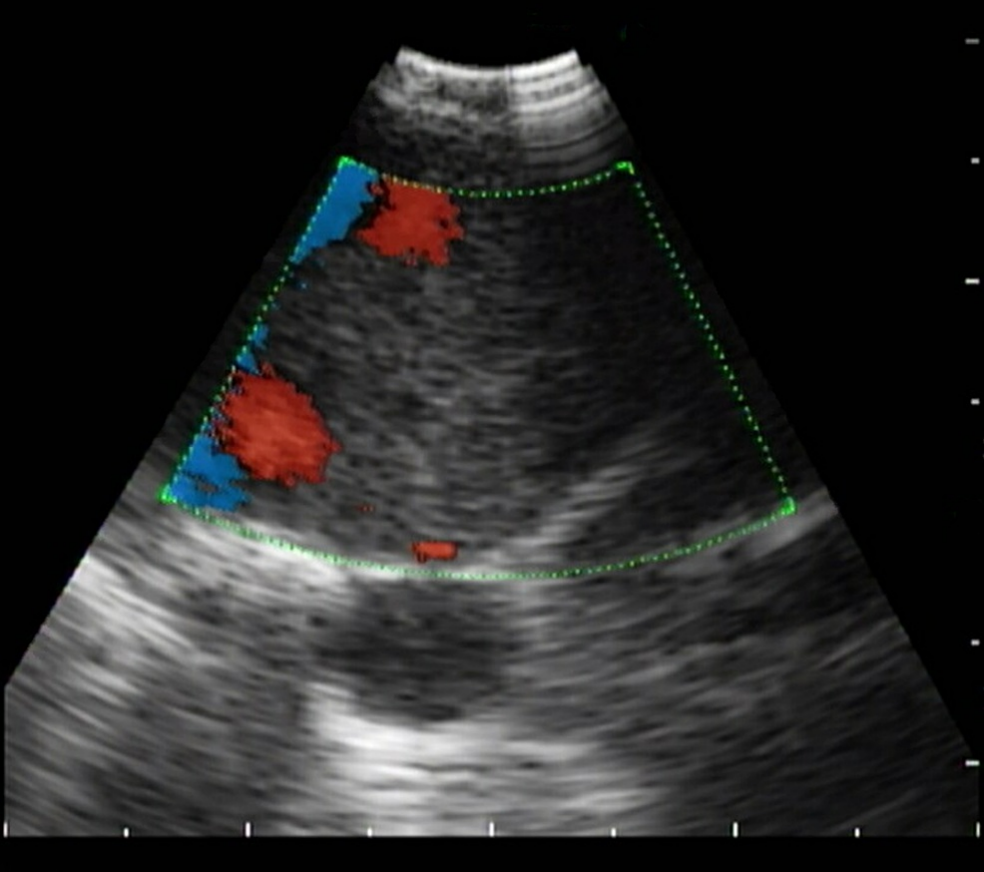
Color Doppler showing the blood flow around the embolus
